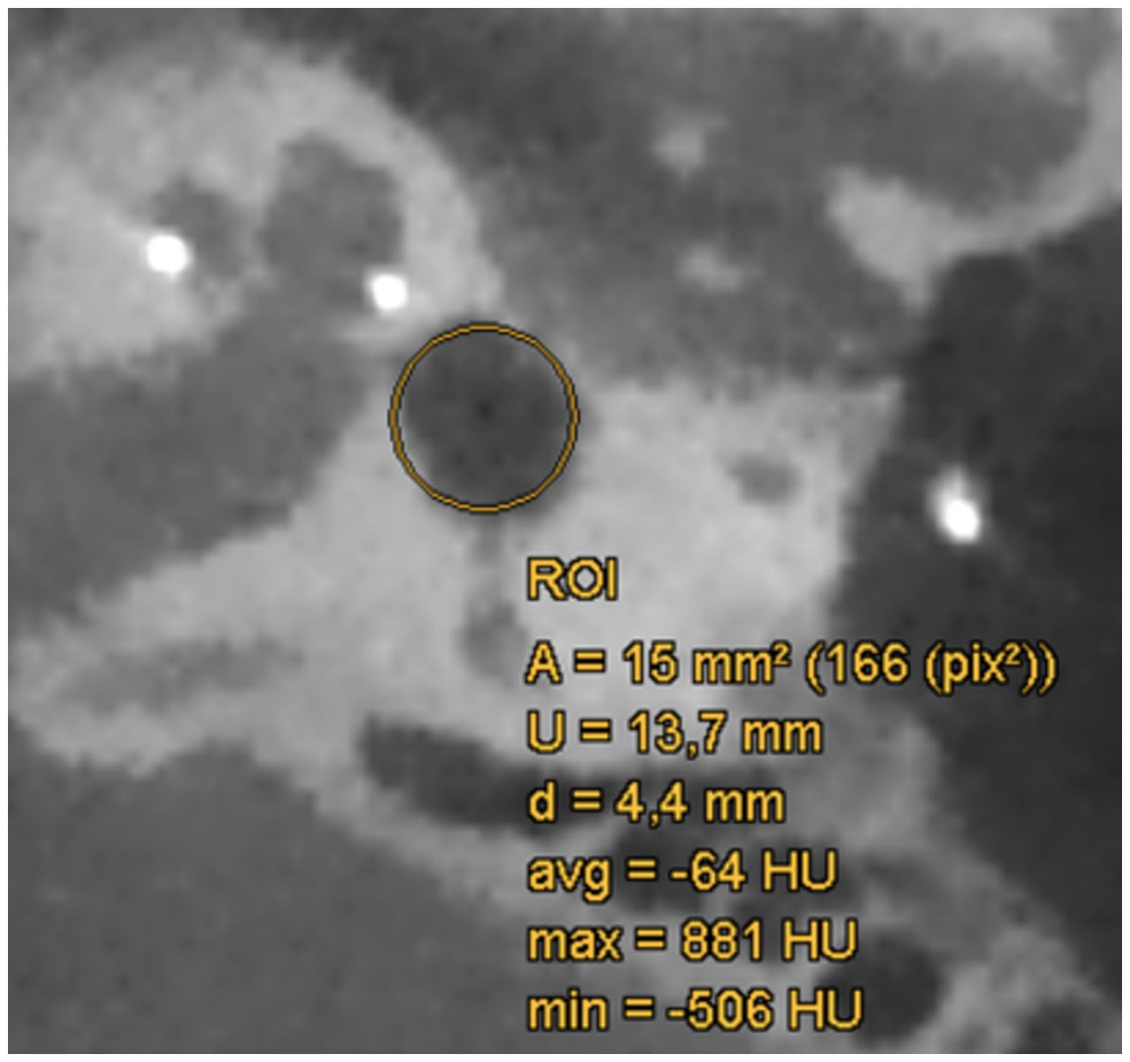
CBCT scan of the labyrinth postoperatively after CI surgery with region of interest (ROI; yellow circle) in the area of the vestibule. The diameter (d) of the ROI is 4.4 mm, the minimal Hounsfield unit (HU) is at - 506 HU.
